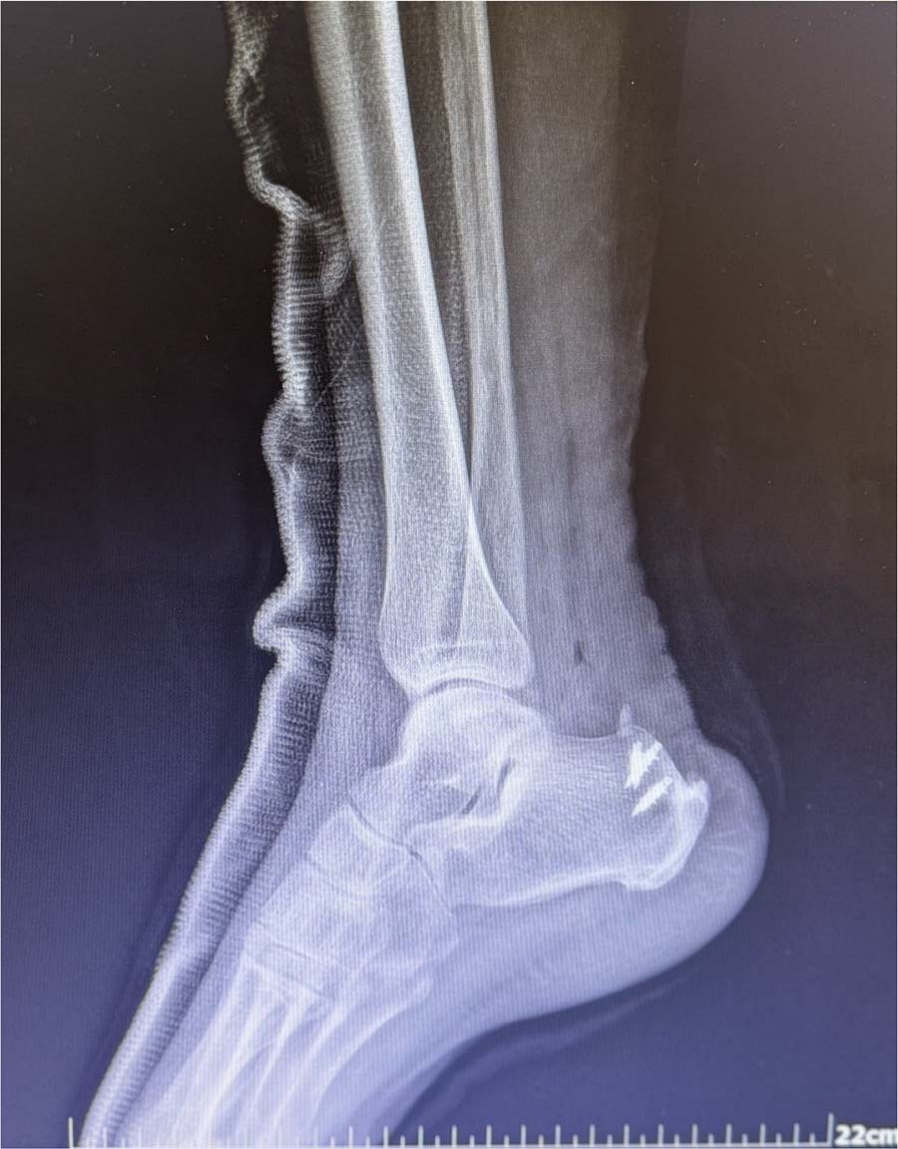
X-ray postoperatively with suture anchors.
